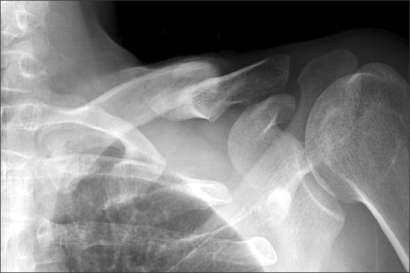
Anteroposterior radiograph of the left clavicle 9 months after the injury. A typical clavicular malunion deformity resulting from that the proximal fragment was displaced superiorly, while the distal fragment was displaced inferiorly, translated medially, and rotated anteriorly after the midshaft clavicular fracture, is demonstrated.
